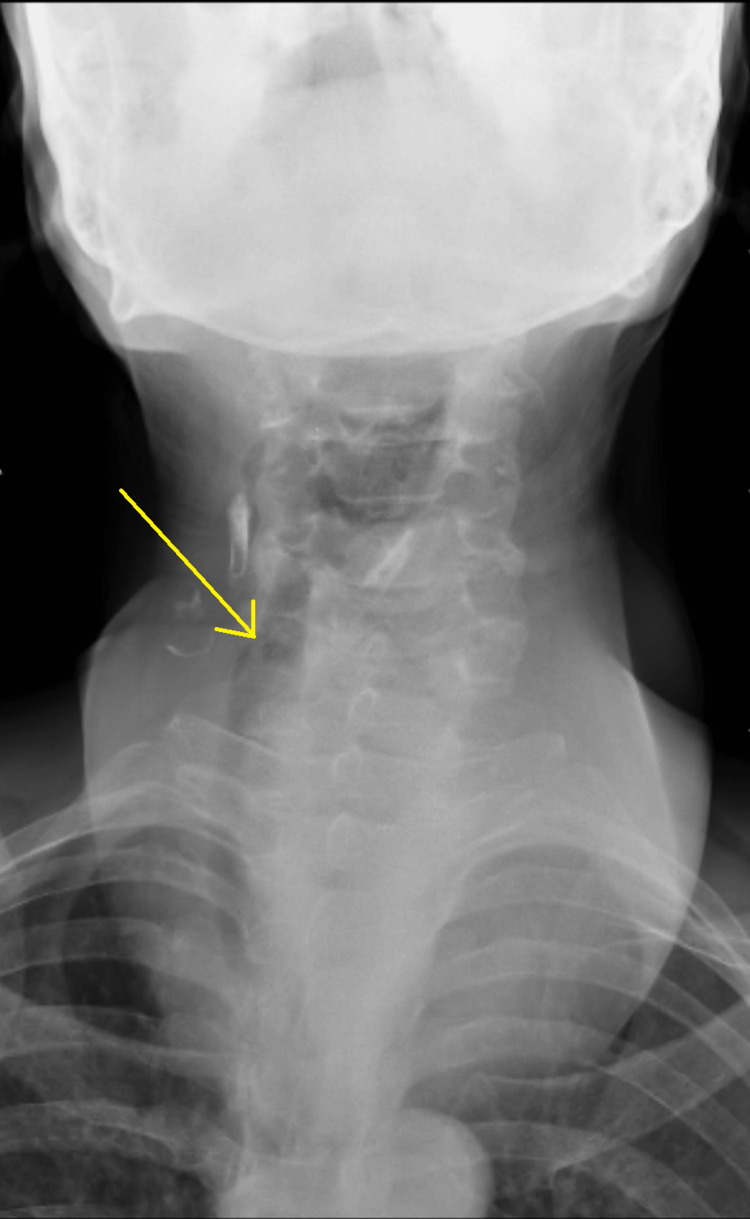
X-ray neck anteroposterior view showing the tracheal deviation
